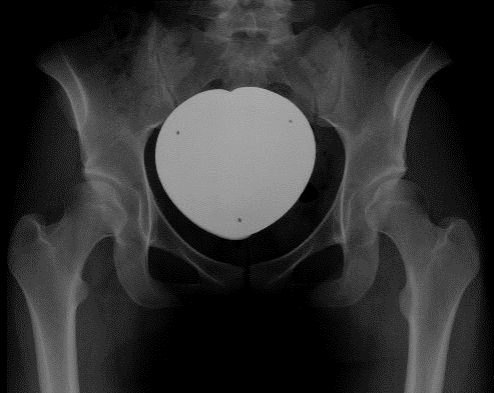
Preoperative radiographic assessment. Indication for surgery included hip pain with the radiological finding of acetabular dysplasia (CE-angle lower than 20 degrees).
